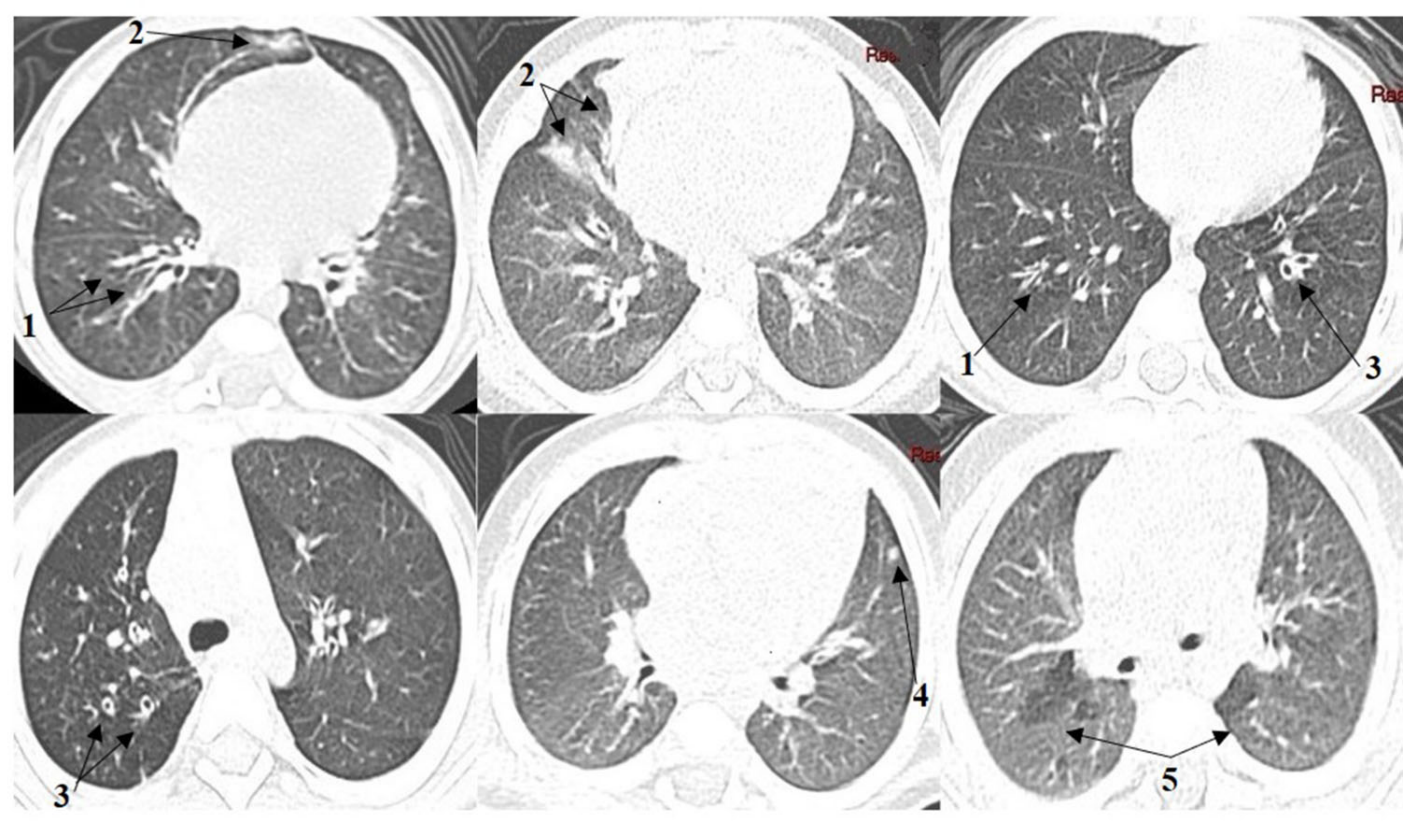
High-resolution computed tomography findings. Bronchial wall thickening (1); Collapse/consolidation (2); Bronchiectasis (3); Mucus plugging (4), Mosaic attenuation/perfusion pattern (5).
